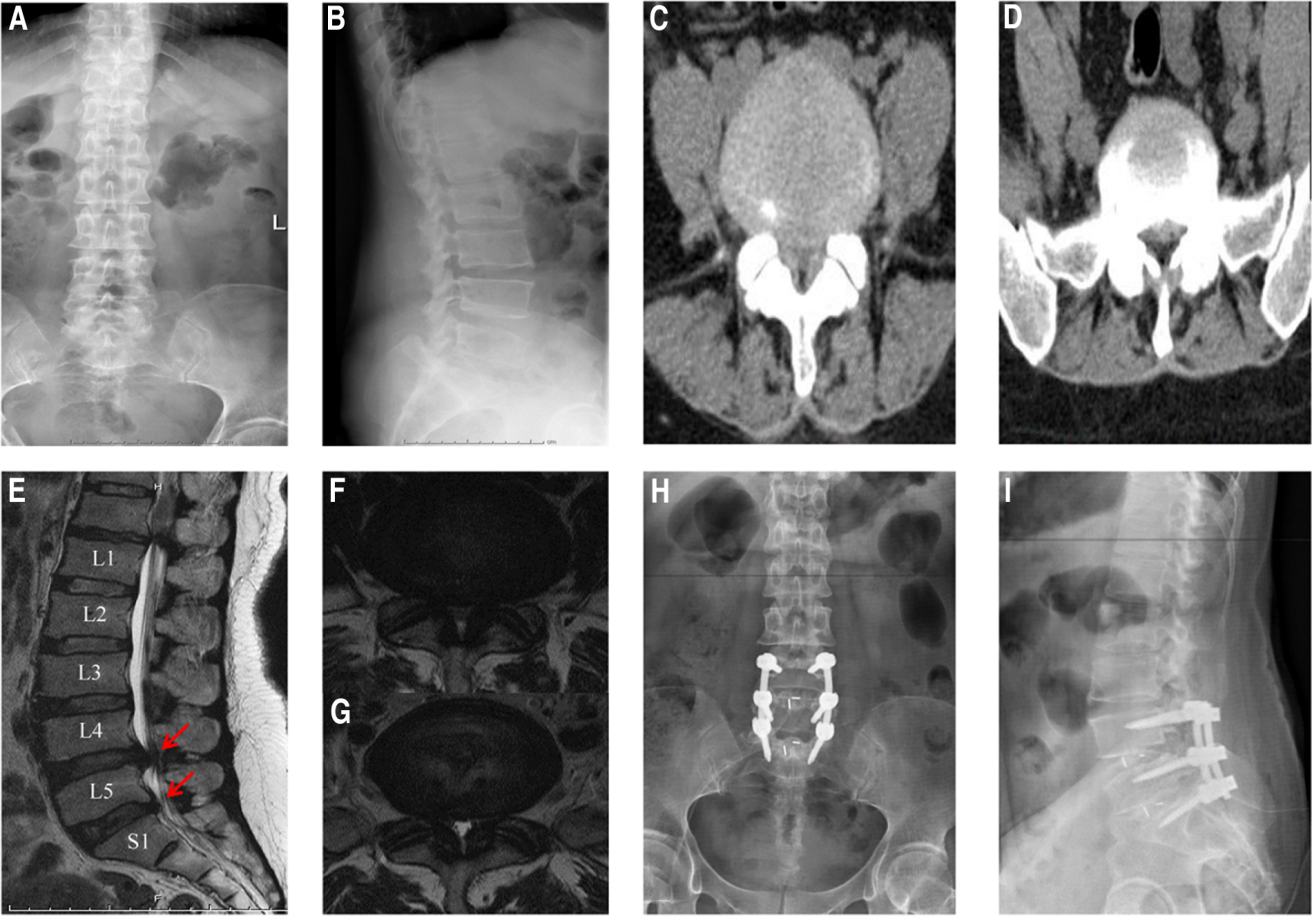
A representative case of posterior fusion surgery. (A,B) Preoperative x-ray radiographs; (C,D) preoperative CT scans of L4-5 and L5-S1, respectively; (E) preoperative MRI scan (sagittal plane); (F,G) preoperative MRI scans of L4-5 and L5-S1, respectively (axial plane); (H,I) postoperative x-ray radiographs. The arrows indicate the herniation of intervertebral disc.
